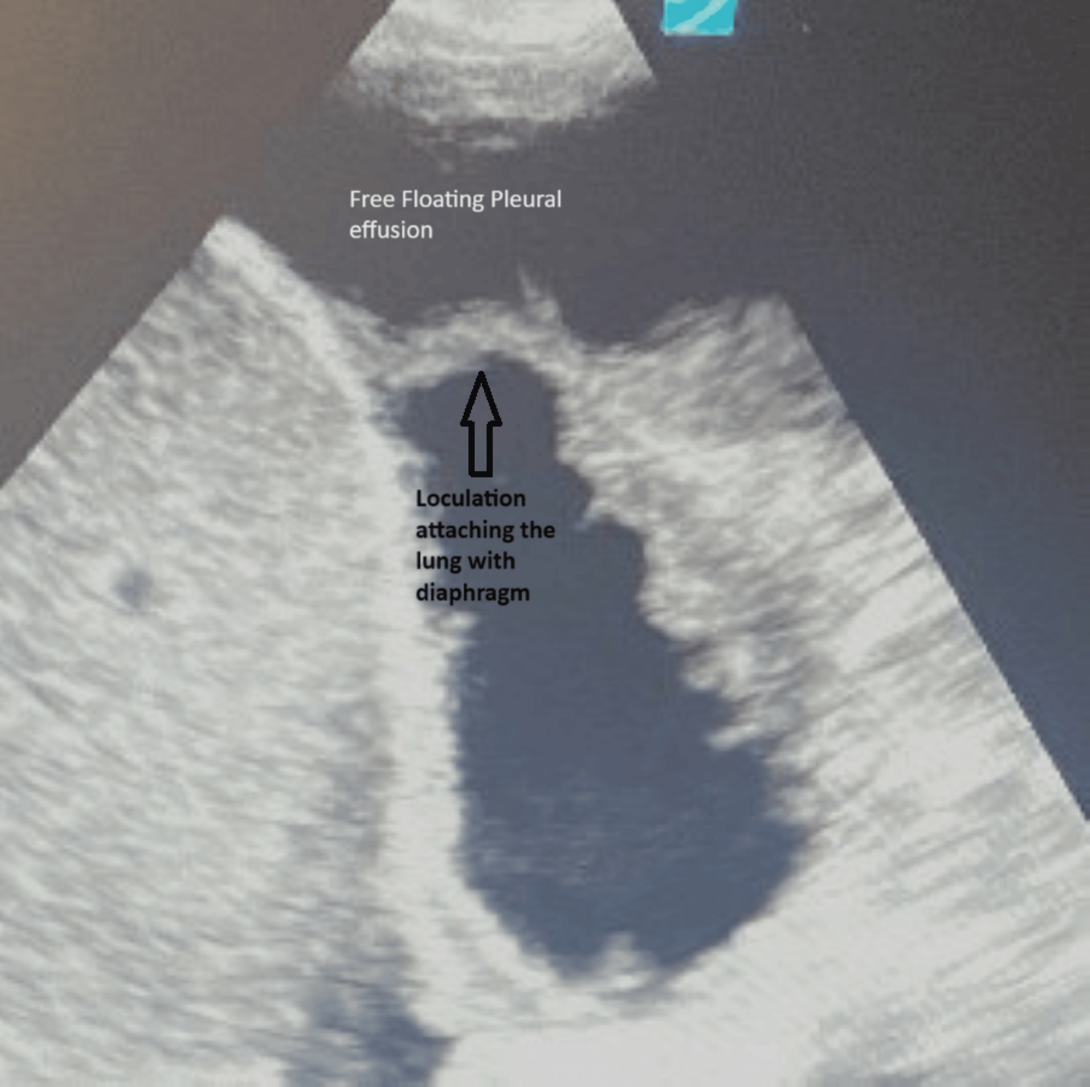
Left-sided lung US showing a fibrin thread (loculations) attached to the diaphragm US: ultrasound
